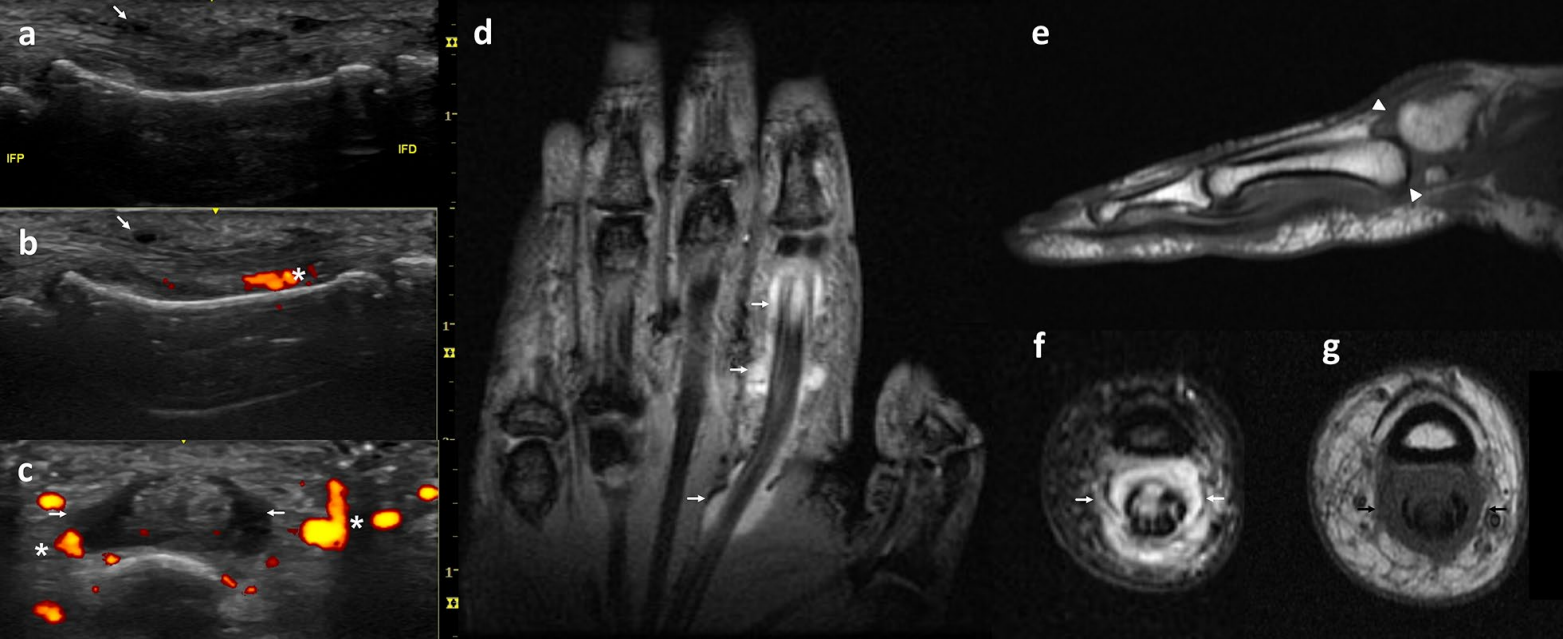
A 48-year-old woman presenting with dactylitis of the second finger of the hand. US examination (a–c) shows a hypoechoic swelling surrounding the flexor digitorum tendons (thin arrow) with Power Doppler signal showing hypervascularity (*) related to tenosynovitis of flexor tendon of the second finger on longitudinal (a, b) and axial (c) views. MR examination adds a general view of the hand in this coronal STIR wi (d) that shows fluid surrounding the second flexor digitorum tendons (thin arrows). Sagittal T1 wi (e) shows subluxation of the second carpo-metacarpal joint (arrow heads). Axial views weigthed on STIR (f) and T1 (g) show extensive tenosynovitis (thin arrows) of the flexor tendons
